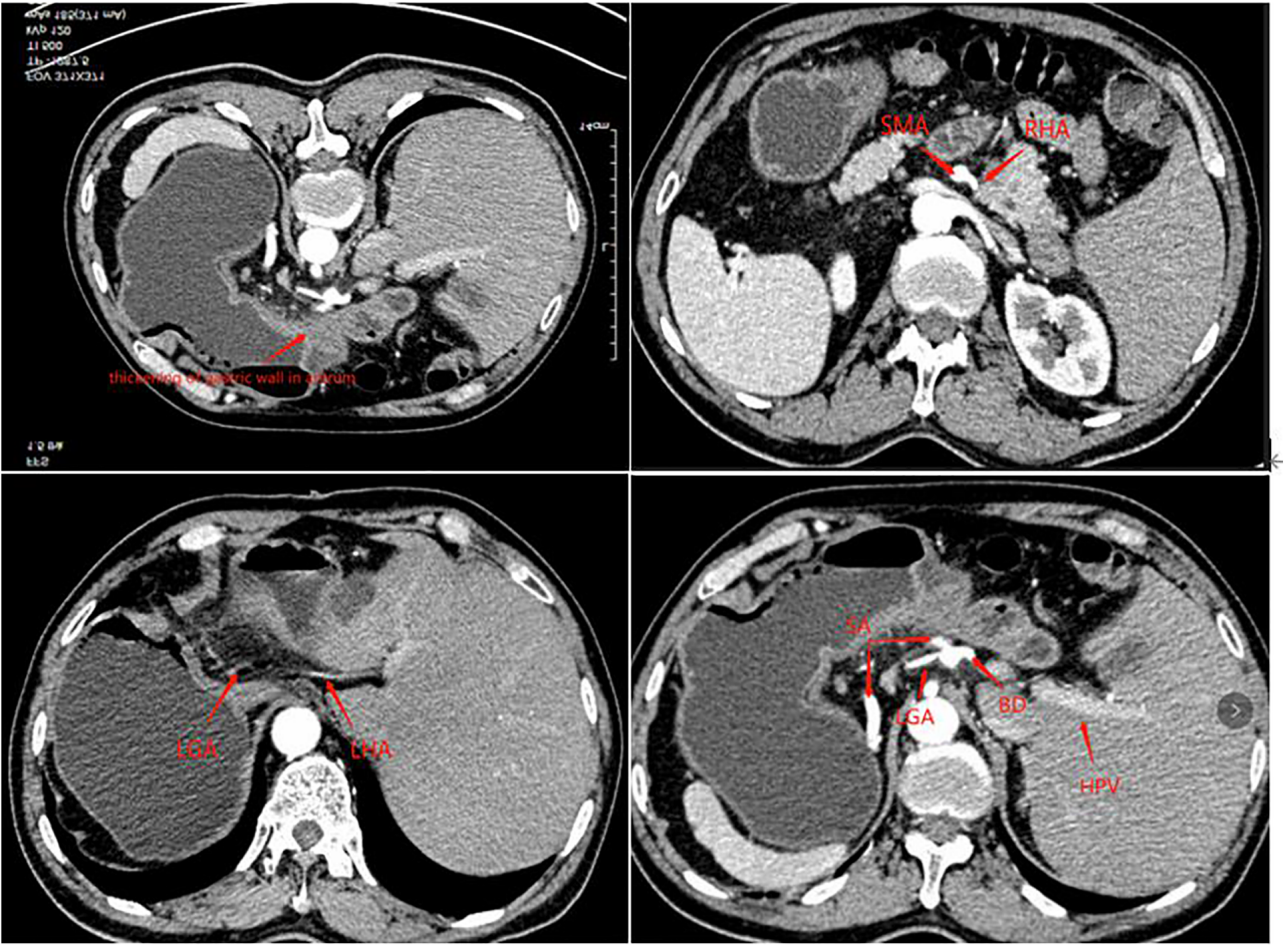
Enhanced CT arterial phase shows vascular variation.
